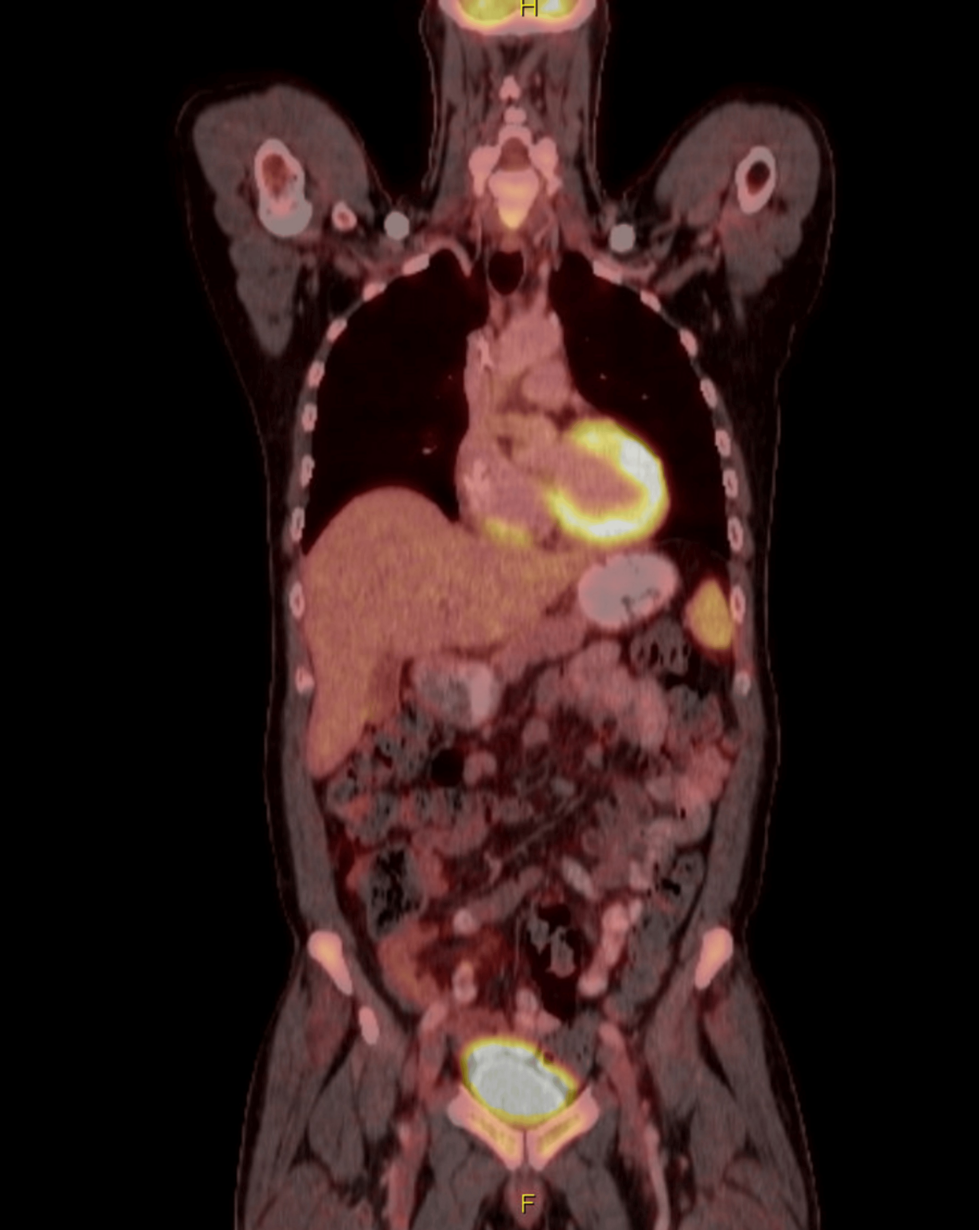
PET-CT after cycle 2 of R-EPOCH chemotherapy Normal physiologic uptake noted in the visualized portions of the myocardium, gastrointestinal tract, and the genitourinary system. No FDG uptake noted in the right atrial infiltrative soft tissue mass. FDG: fluorodeoxyglucose, PET-CT: positron emission tomography-computed tomography, R-EPOCH: rituximab, etoposide, prednisone, vincristine, cyclophosphamide, and doxorubicin.
